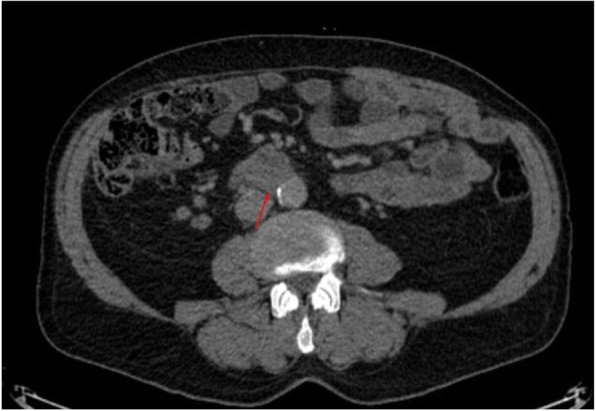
Radiological progression of disease with paraaortic mass, enlarged to 32 × 28 mm in computed tomography axial view
